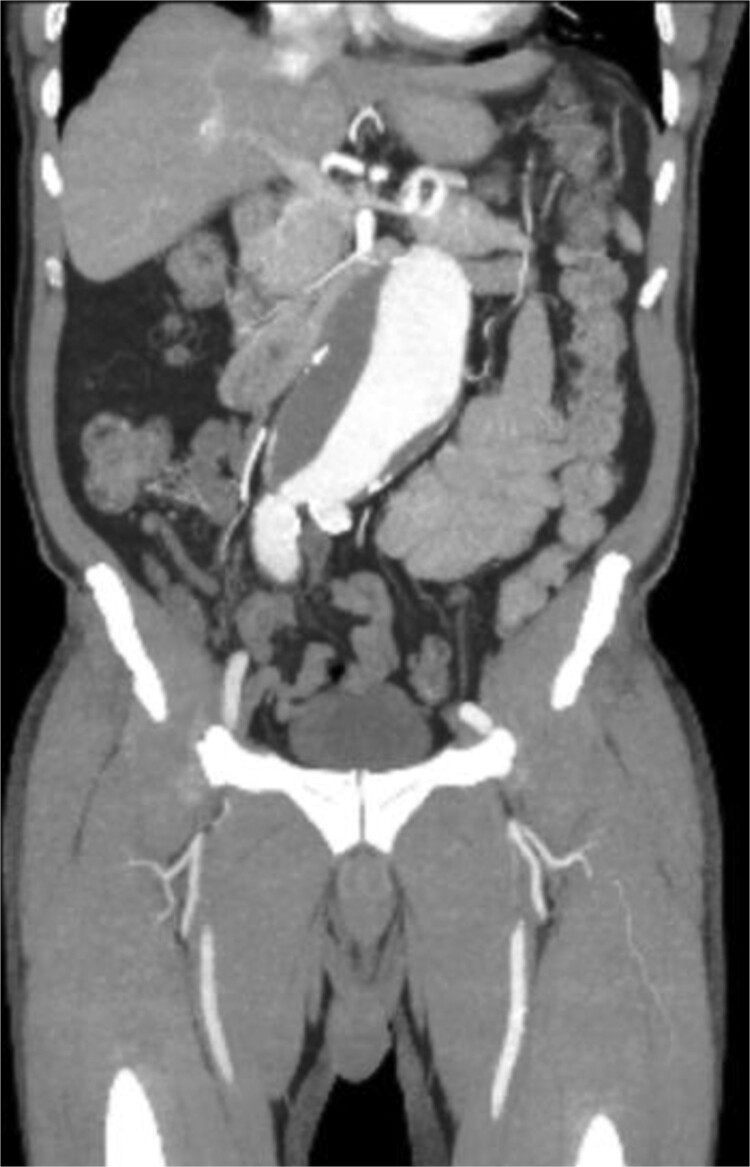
CT angiogram of abdominal aorta and iliofemoral artery coronal view.
